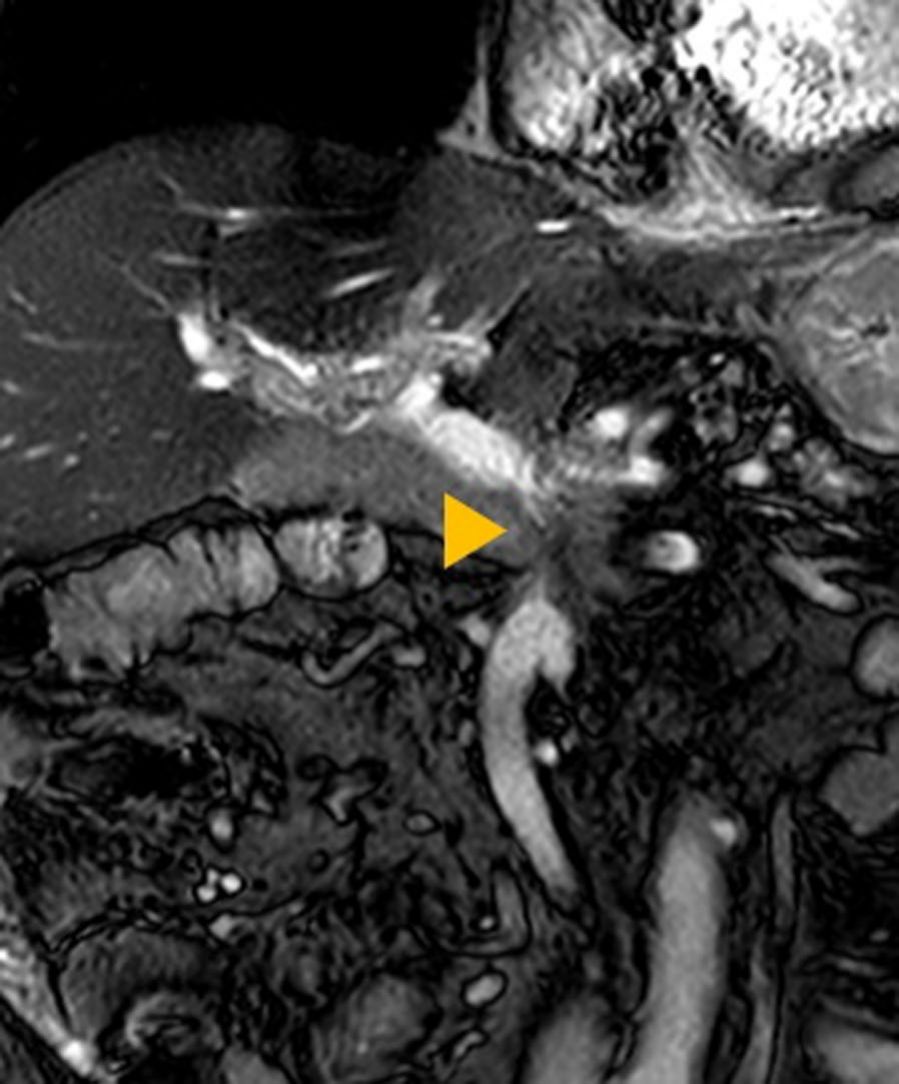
Preoperative findings of contrast-enhanced Magnetic Resonance Imaging (coronal image). The superior mesenteric vein was obstructed 2 cm near the splenic vein confluence (arrowhead), and the splenic vein was stenotic
